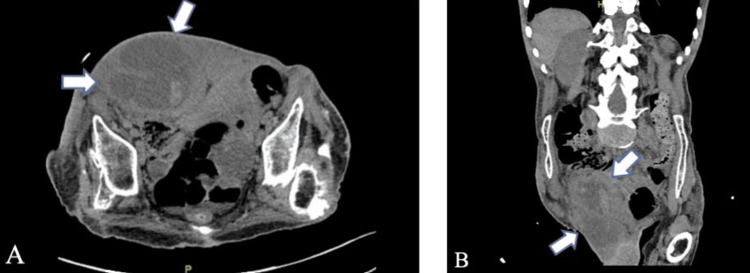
Transverse (A) and coronal (B) views on CTAP without contrast showing 10 x 10 x 20 cm collection (arrow) in the right anterior lateral pelvis centered upon the right rectus sheath with irregular peripheral enhancement extending into the right inguinal region and scrotum. CTAP: CT abdomen and pelvis
